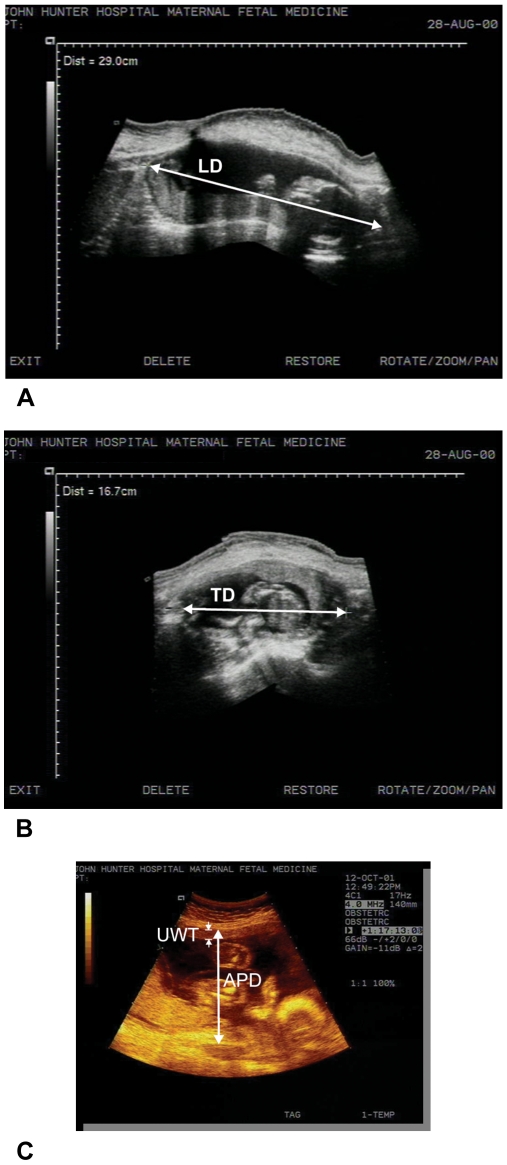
Examples of ultrasonographic measurements taken with an Aspen Advanced Ultrasound System. (A) Longitudinal Diameter (LD) of the uterus using Freestyle™ software; (B) Transverse Diameter (TD) of the uterus using Freestyle™ software; (C) Anterior-Posterior Diameter (APD) and Uterine Wall Thickness (UWT) shown.
